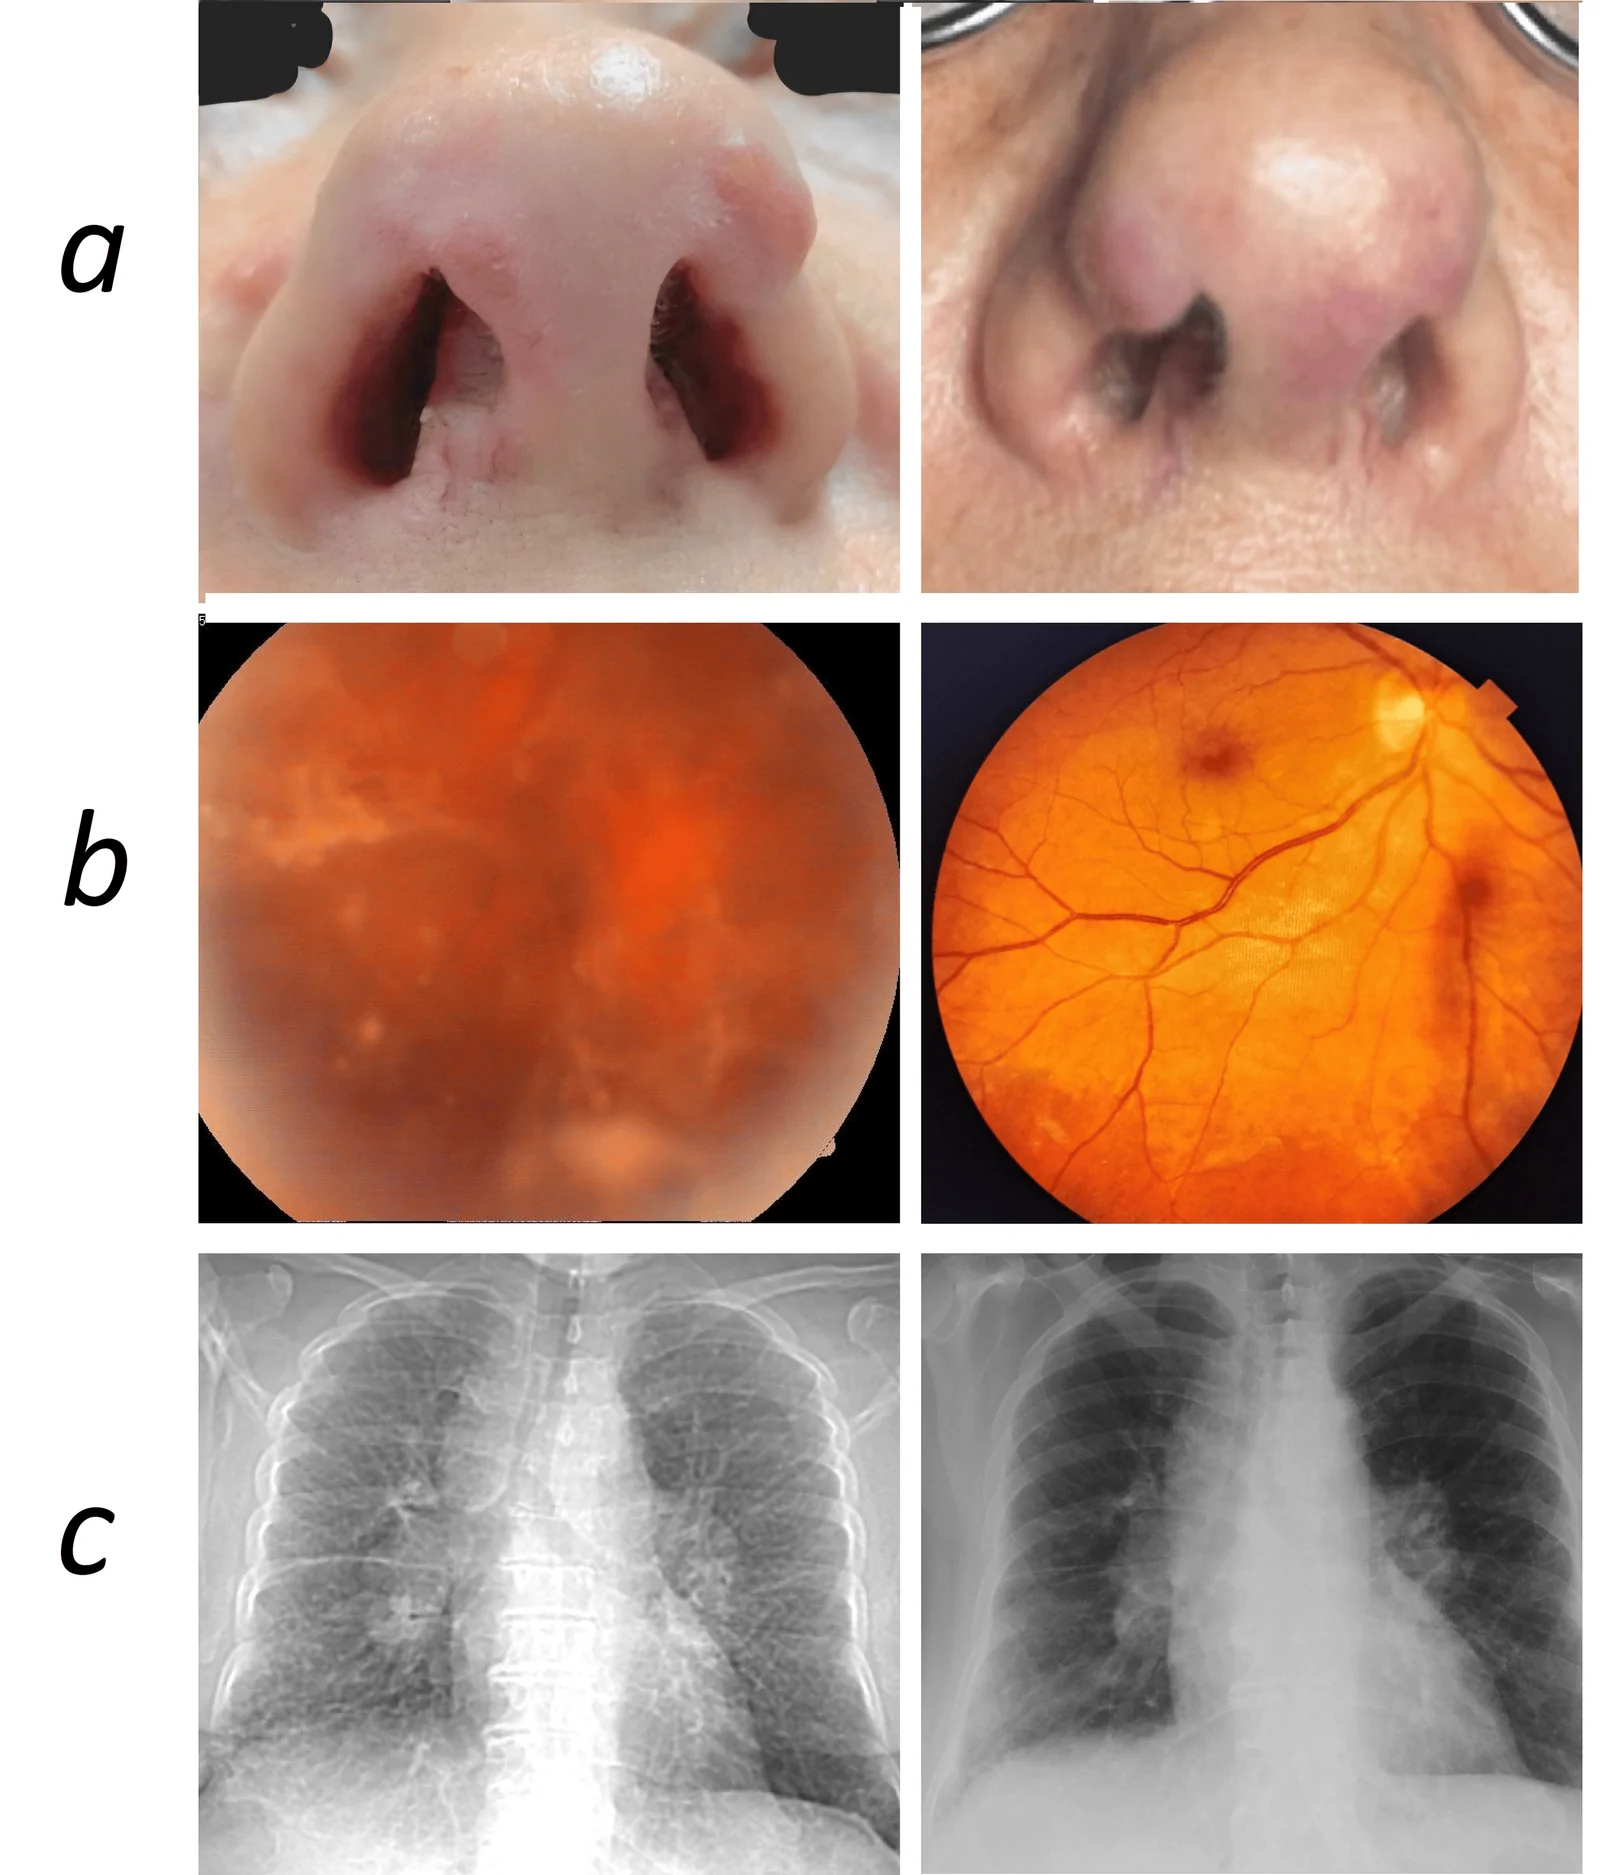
Clinical progress before (left) and one month after (right) systemic corticosteroid therapy a. Nasal nodules. b. Fundoscopy of the left eye showing the resolution of the yellowish-white vitreous opacities suspended. c. Chest X-ray demonstrating a significant decrease in hilar lymphadenopathy.
